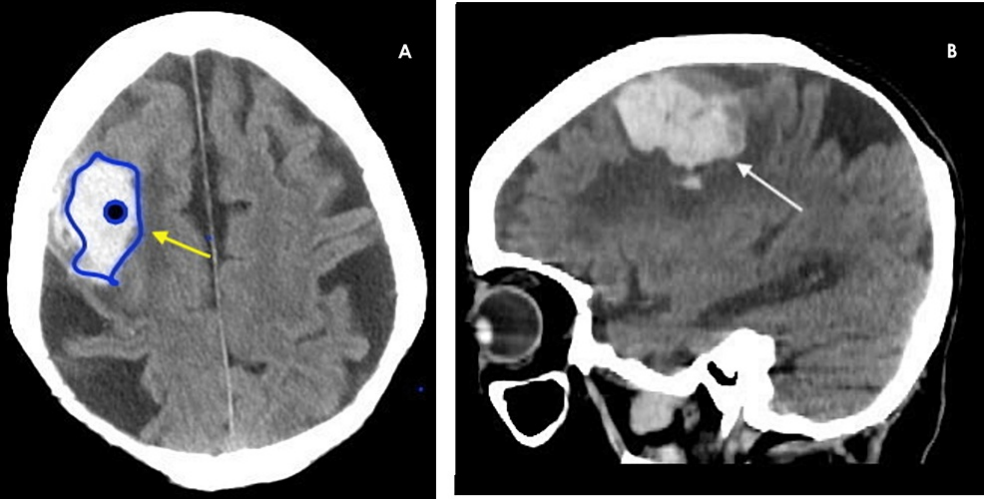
Axial (panel A) and sagittal (panel B) views of brain CT scans demonstrating right-sided intracerebral hemorrhage (ICH) located around the frontal and parietal lobes (arrow)
